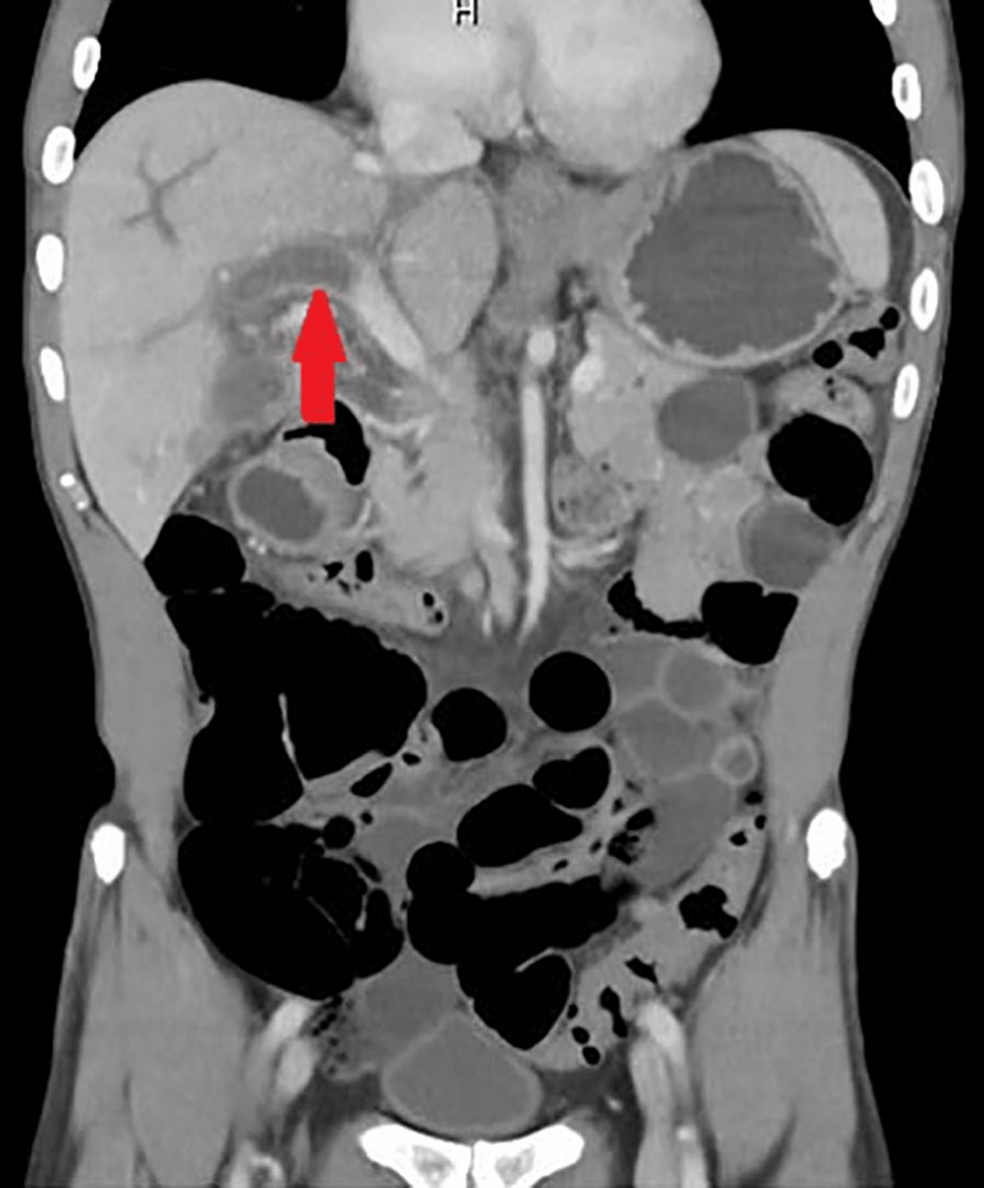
CT of the abdomen and pelvis pointing at portal vein thrombosis.
